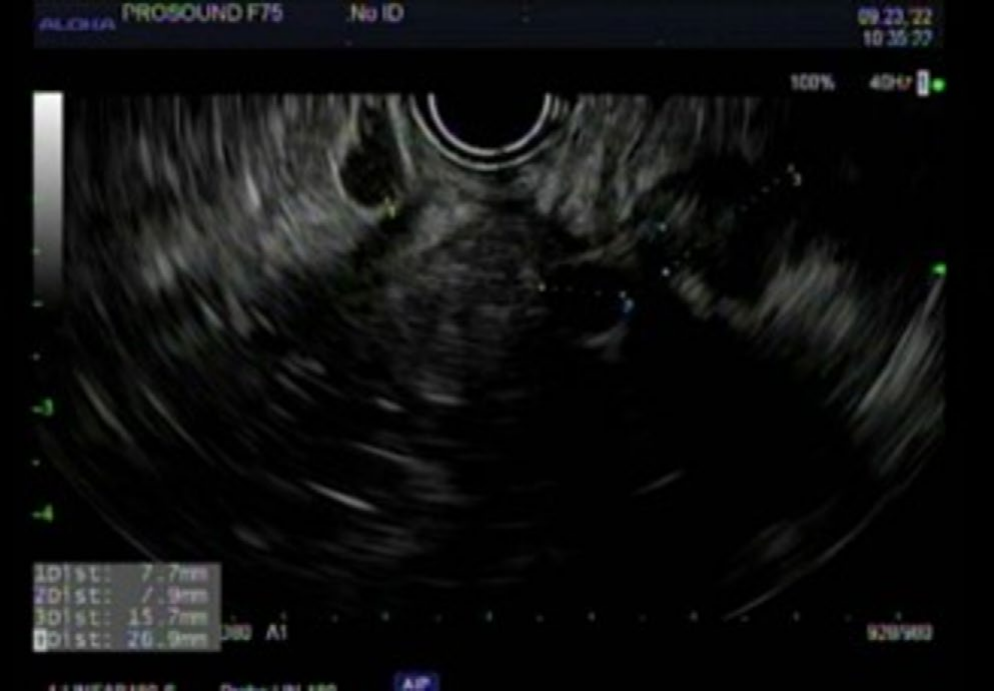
Endoscopic ultrasound findings of recurrence of tumor at site of previous scar.
